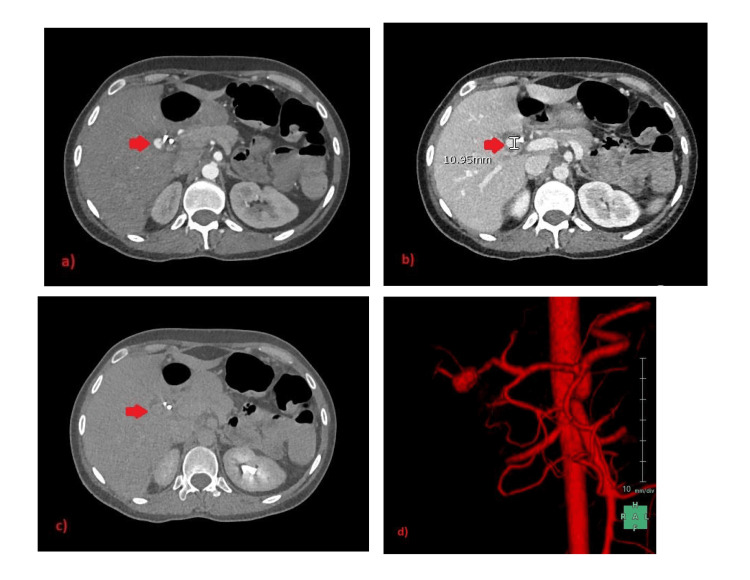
Selected images of computed tomography abdomen angiogram showing cystic artery stump pseudoaneurysm. Axial images of computed tomography (CT) abdominal angiogram study representing arterial (a), venous (b), and delayed acquisition phases (c), respectively, showing focal contrast-filled outpouching (arrows) at the porta hepatis along the lateral aspect of right hepatic artery closely related to cholecystectomy clip and biliary stent. The lesion appears iso-dense to the aorta on arterial phase image (a), and venous phase (b) and washing-out on a delayed scan (c), corresponding to a cystic artery stump pseudo aneurysm. (d) 3D reconstruction coronal view of the arterial phase on CT abdomen showing the origin of cystic artery stump pseudo aneurysm.
